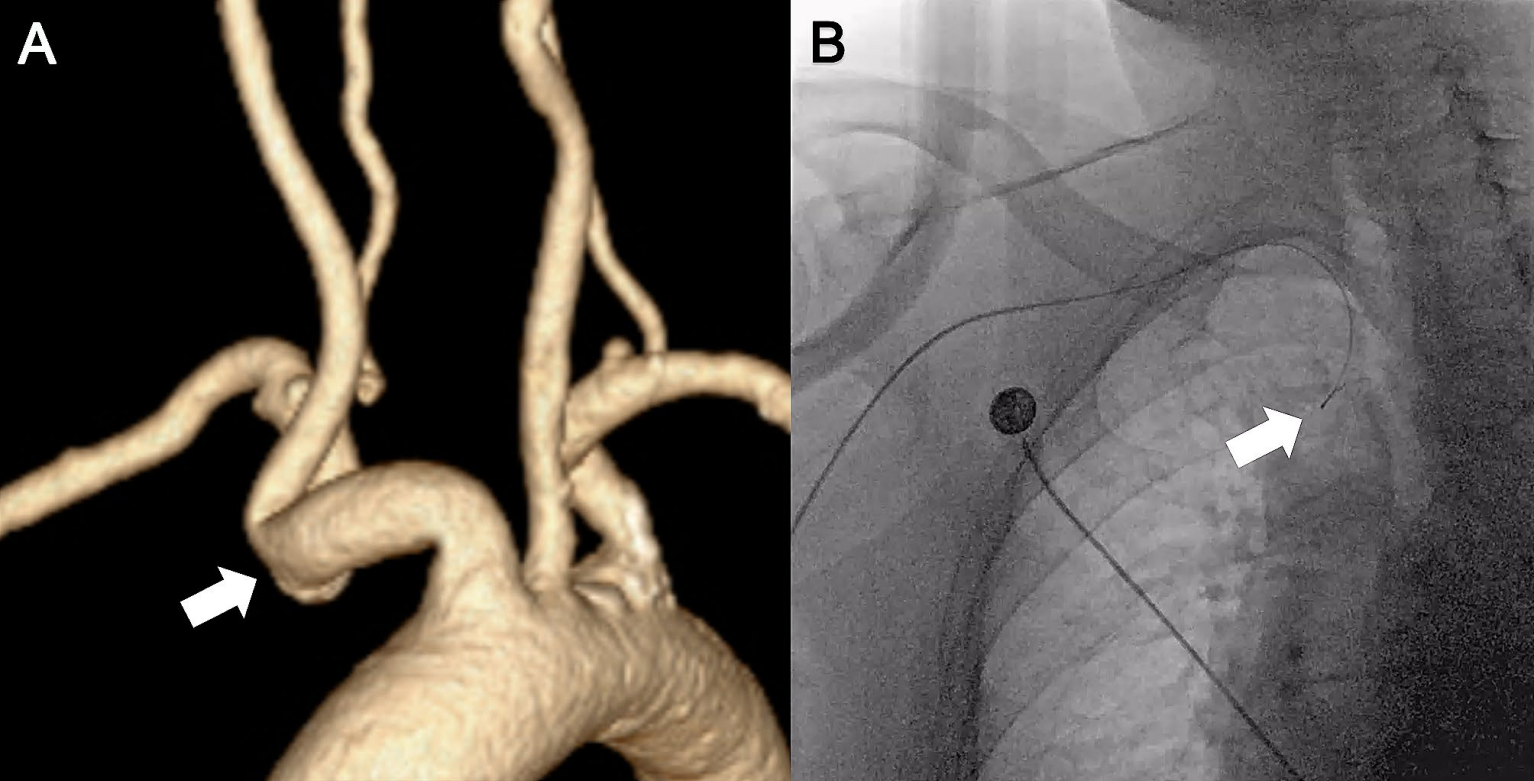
Demonstration of tortuous subclavian artery, making it difficult for the guide wire to pass through. (A) Computed tomography angiography. (B) Trans radial cerebral angiography
